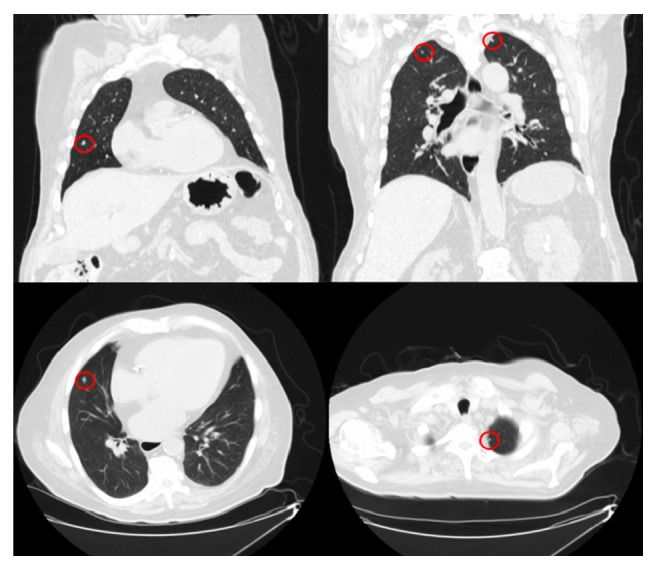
Computed tomography (CT) scan of the chest revealed multiple bilateral pulmonary and pleural-based nodules* (red circles)* suggestive of metastasis.
